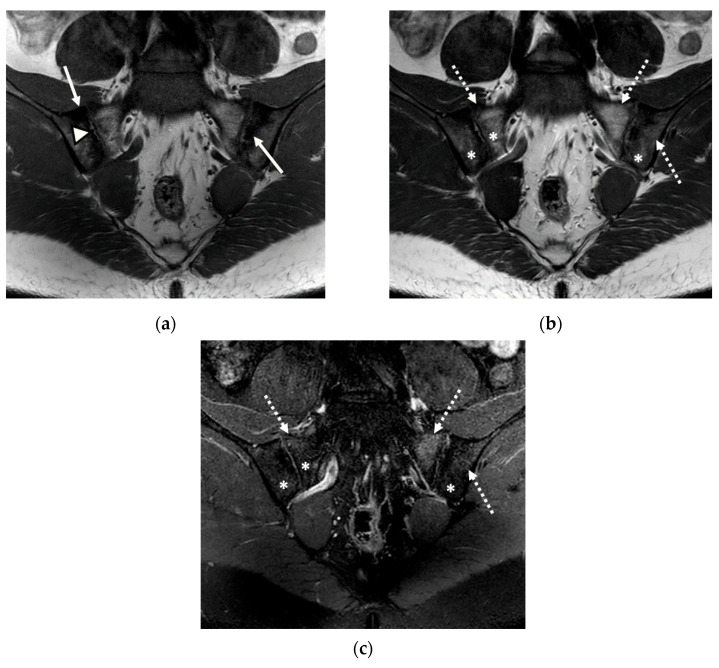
The MRI scan of the sacroiliac joints indicated bilateral narrowing of the sacroiliac joint space, associated with bilateral subchondral sclerosis of the iliac bones (continuous arrow); bilateral fat metaplasia (asterisk), mostly affecting the iliac bones and the right part of the sacrum; backfill of the right sacroiliac joint space (arrowhead); diffuse bone marrow edema (discontinuous arrow), affecting the sacrum and the left iliac bone: (a) T1-weighted sequence; (b) T2-weighted sequence (without fat suppression); (c) STIR sequence.
